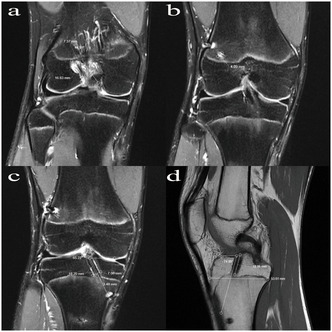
Right Knee. Postoperative magnetic resonance imaging at 3‐month follow‐up showing consistency between the actual bone tunnel trajectory and the preoperatively planned individualized tunnel pathway.
